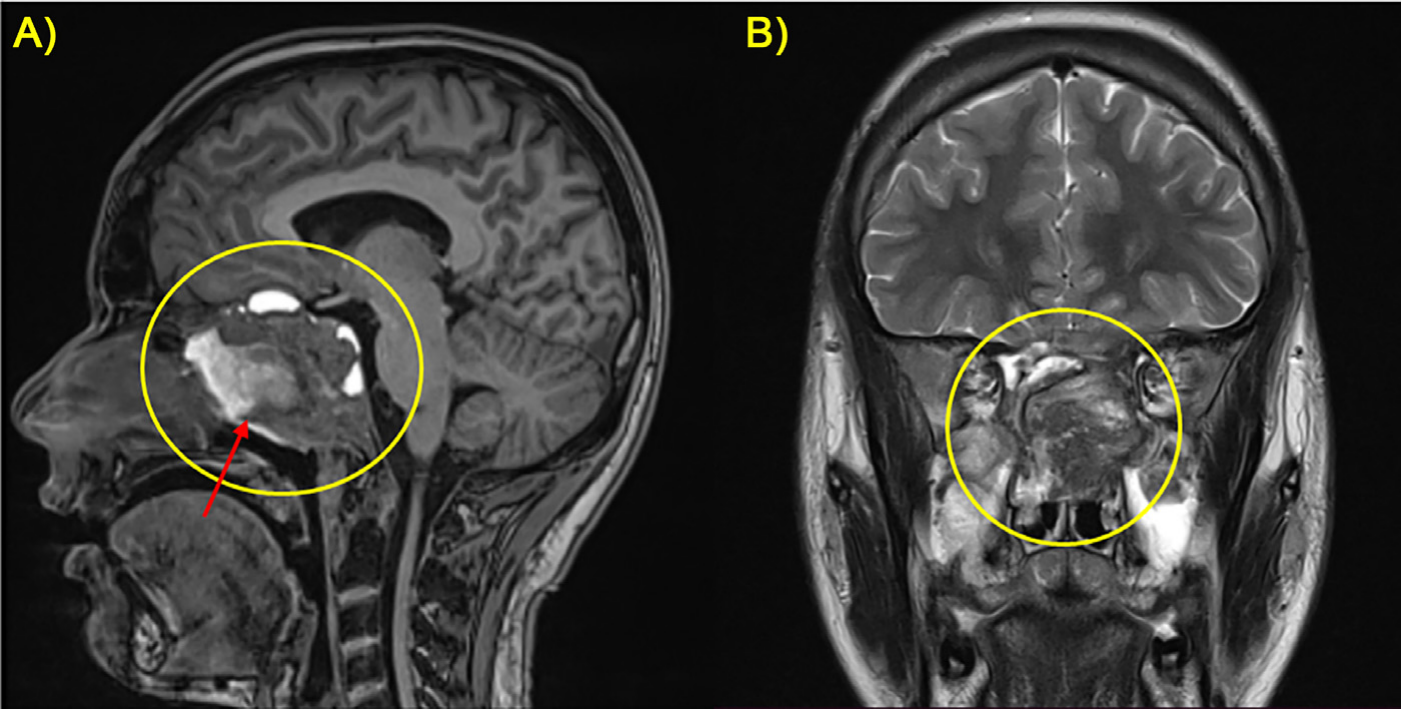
(A, B) Postoperative MRI with sagittal FLAIR and axial T2 sequences showed a voluminous heterogeneous residual mass (yellow circle) with necrotic areas (red arrow).
